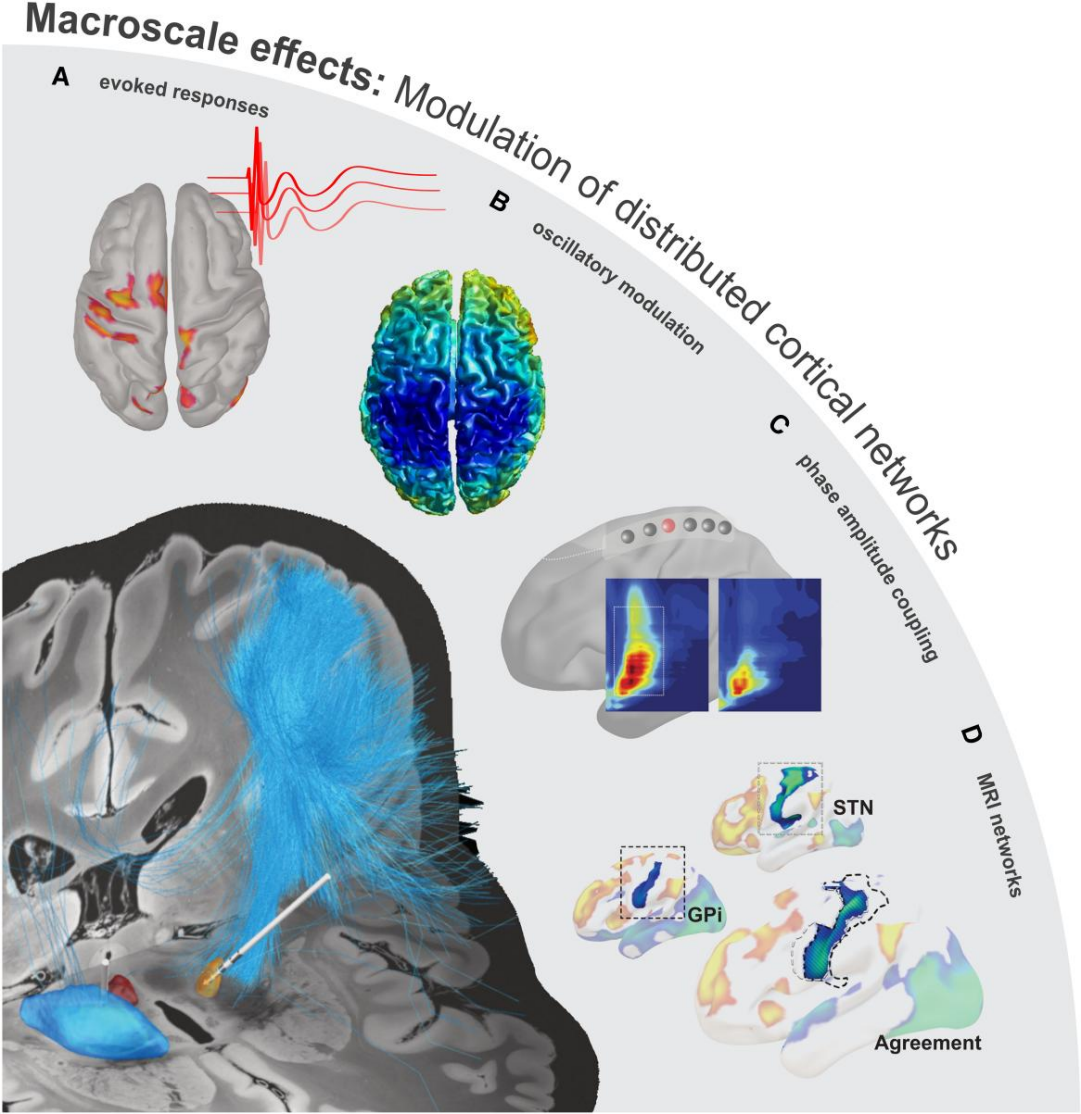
Macroscale effects of DBS. In addition to neurophysiological circuit alterations at the local level (i.e. subcortical functional changes at target structures), deep brain stimulation (DBS) has an influence upon whole-brain interregional networks. The modulation of a single node of a circuit will likely lead to state changes in all circuit connections. This could, in part, be achieved by local axonal activations (*bottom left*), as evidenced by (A) short-latency stimulation-induced evoked potentials in the cortex^128,129^; wherein the strength of network engagement (evoked potential amplitude) may be reflective of the achieved anti-parkinsonian efficacy with therapeutic settings (adapted from Bahners *et al*.^128^). Moreover, in addition to the suppression of subcortical beta oscillations, (B) DBS can suppress beta power across the sensorimotor cortical network (adapted from Abbasi *et al*.^109^), as well as (C) attenuate cortico-cortical beta-gamma coupling (adapted from De Hemptinne *et al*.^112^), which is otherwise pathologically elevated in Parkinson’s disease.^110^ Despite such findings, direct antidromic activation of cortical circuitry is suggested not be able to corroborate the clinical efficacy of GPi-DBS.^5^ (D) As such, the spatial overlap in functional connectivity, which co-localizes the predictive efficacy of STN- and GPi-DBS to the M1 region may be representative of a slower timescale (functional MRI-based) functional readout of a downstream basal-ganglia-thalamo-cortical network effect, which could be generated by a convergent GPe-mediated subcortical network activation profile achieved by STN- and GPi-DBS (i.e. ERNA). GPe = globus pallidus externus; GPi = globus pallidus internus; STN = subthalamic nucleus.
